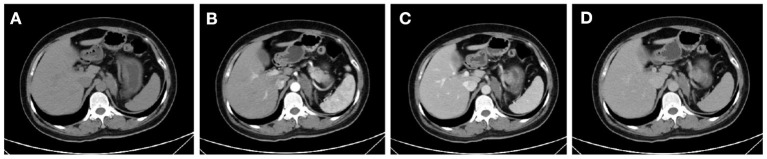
CT showed an irregular mass of soft-tissue density above the left kidney in the plain scan (A). In enhanced scan, the arterial phase (B), venous phase (C), and delayed phase (D) showed uneven and mild enhancement.
